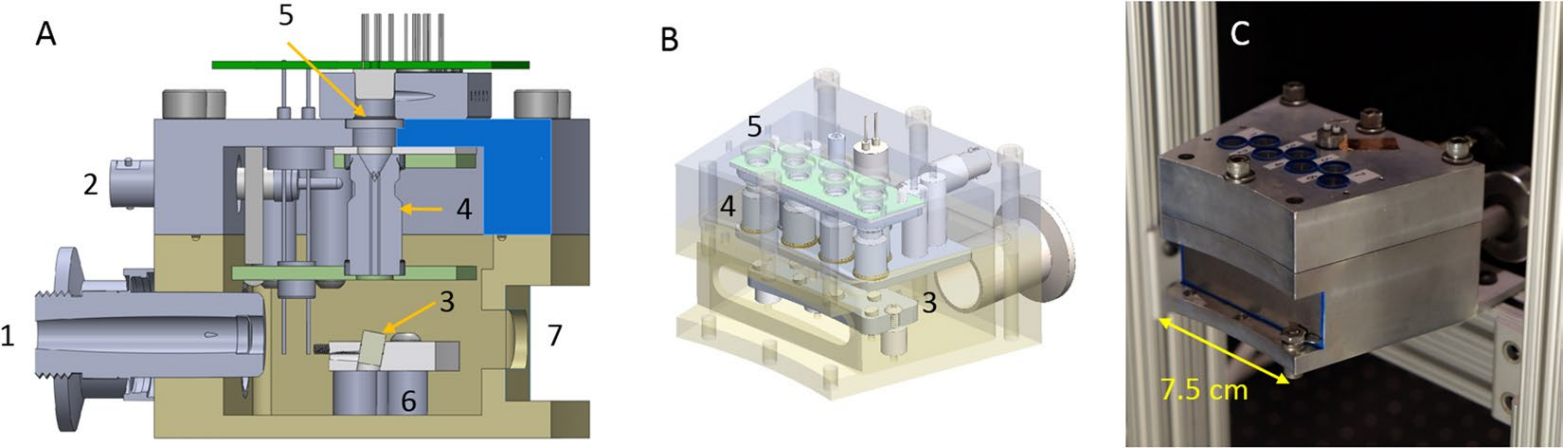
(A) Cut-through CAD model of the module. (B) Isometric view with a transparent outer housing. (C) Completed x-ray module with a beryllium sheet covering the x-ray window.(1) Vacuum connector (2) 3 kV bias line (3) tungsten targets (4) Channeltron electron amplifiers (5) quartz windows (6) High-voltage anode plate (7) x-ray window.
